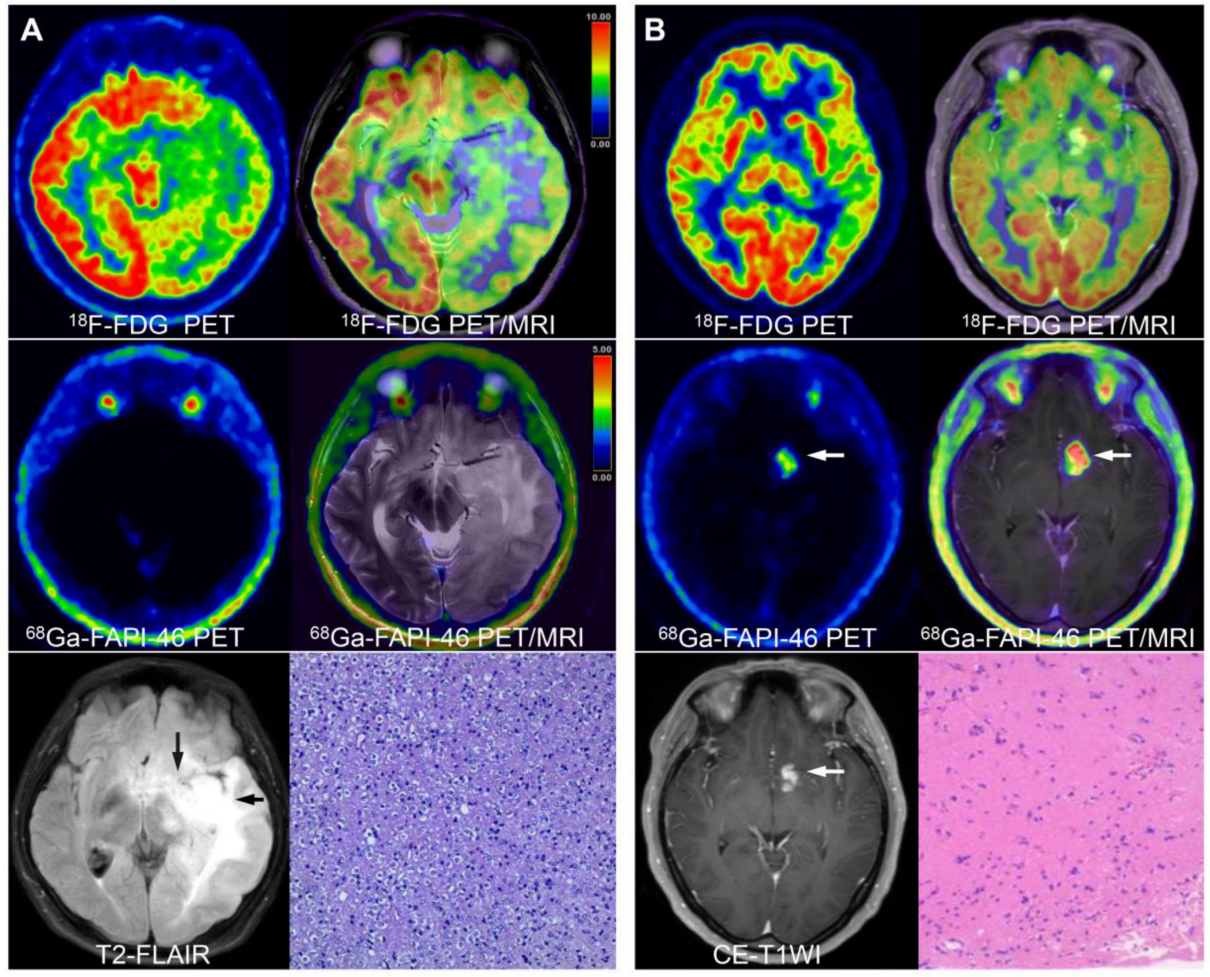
True-positive and false-negative findings of low-grade gliomas on ^68^Ga-FAPI-46 PET (lesions are indicated by arrows). A 17-year-old male presented with recurrent vomiting. No significant tracer uptake was observed on ^68^Ga-FAPI-46 PET. ^18^F-FDG PET showed diffusely reduced glucose metabolism in the left cerebral parenchyma. T2-FLAIR images revealed abnormal high signals in the left temporal lobe, basal ganglia, insula, thalamus, and brainstem. The final pathological diagnosis was diffuse astrocytoma (A). A 38-year-old female presented with a headache. No significant abnormalities were observed on ^18^F-FDG PET. However, on ^68^Ga-FAPI-46 PET and PET/MRI fusion images, intense radiotracer uptake was observed in the left basal ganglia, which showed abnormal enhancement on CE-MRI. The final pathological diagnosis was a low-grade ganglioglioma (B).
